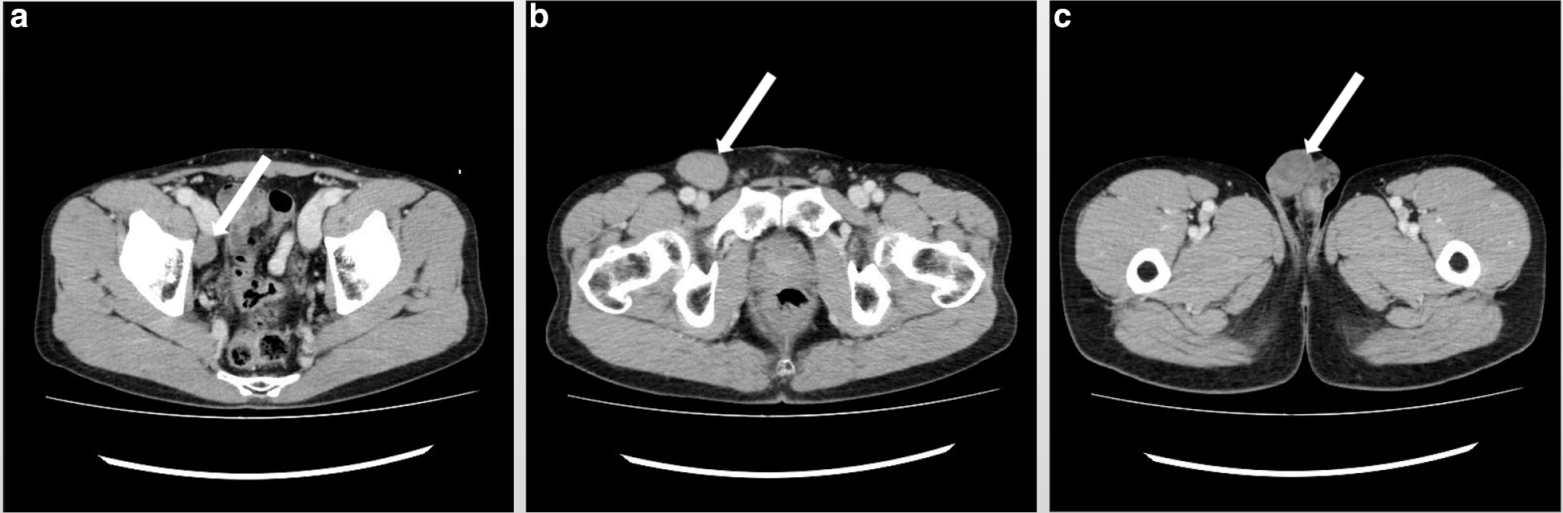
Contrast-enhanced pelvic CT. a Enlarged right external iliac lymph node (white arrow). b Enlarged right inguinal lymph node (white arrow). c Enhanced right testicular tumor (white arrow). The diagnosis was testicular tumor (cT1, N2, M0, S0, and TNM stage IIB)
